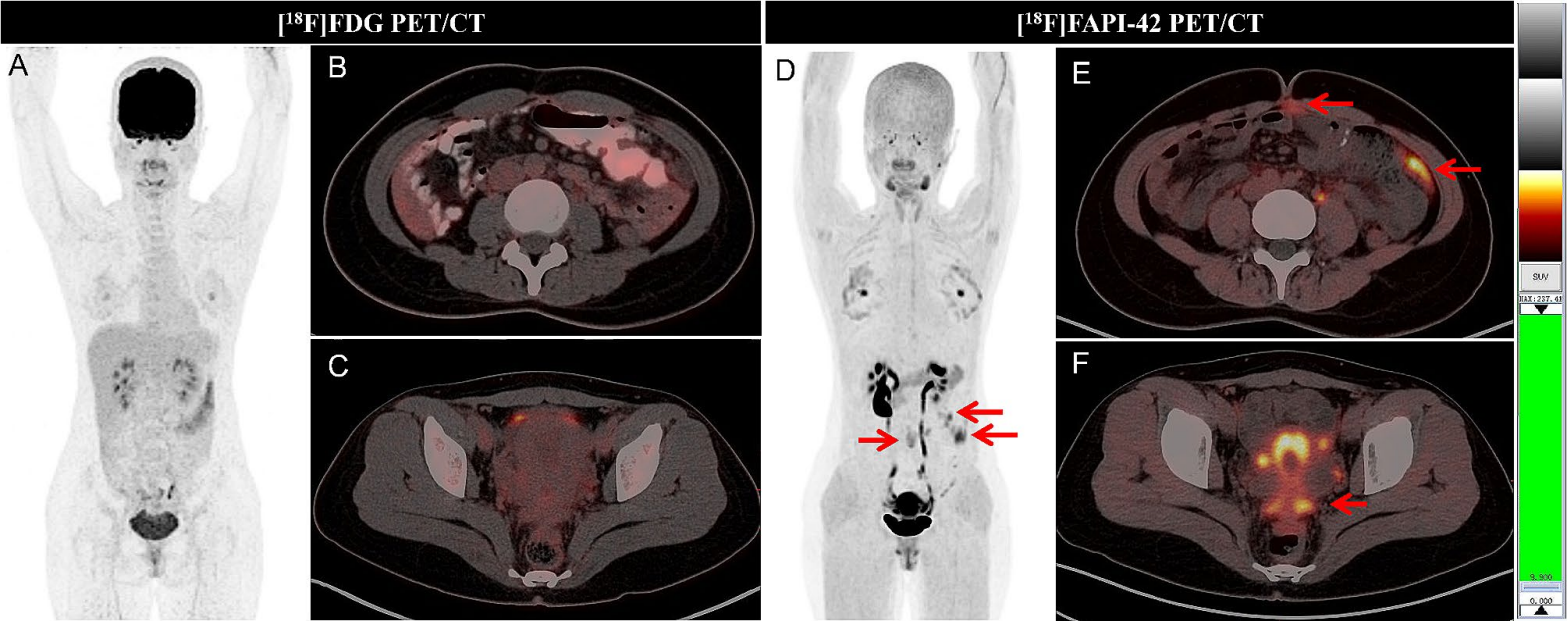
A 29-year-old woman had a medical history of surgery of goblet cell adenocarcinoma for 2 years and without discomfort. She underwent PET/CT scan for regular follow-up. However, no positive was observed on ^18^F-FDG PET MIP(A) and axial fused images (A ∼ C). However, the MIP of ^18^F-FAPI-42 PET showed mild to intense FAPI uptake in the middle and left abdominal (D, red arrows), the axial fused images of ^18^F-FAPI-42 PET/CT showed FAPI was taken up by the lesions in the left abdominal omentum majus (SUVmax, 3.4 ~ 5.7, E, red arrows) and utero-rectal recess (SUVmax, 8.2, F, red arrow).^18^F-FAPI-42 PET/CT is superior to ^18^F-FDG PET/CT in monitoring tumor recurrence in goblet cell carcinoma patient and changed the treatment plan
